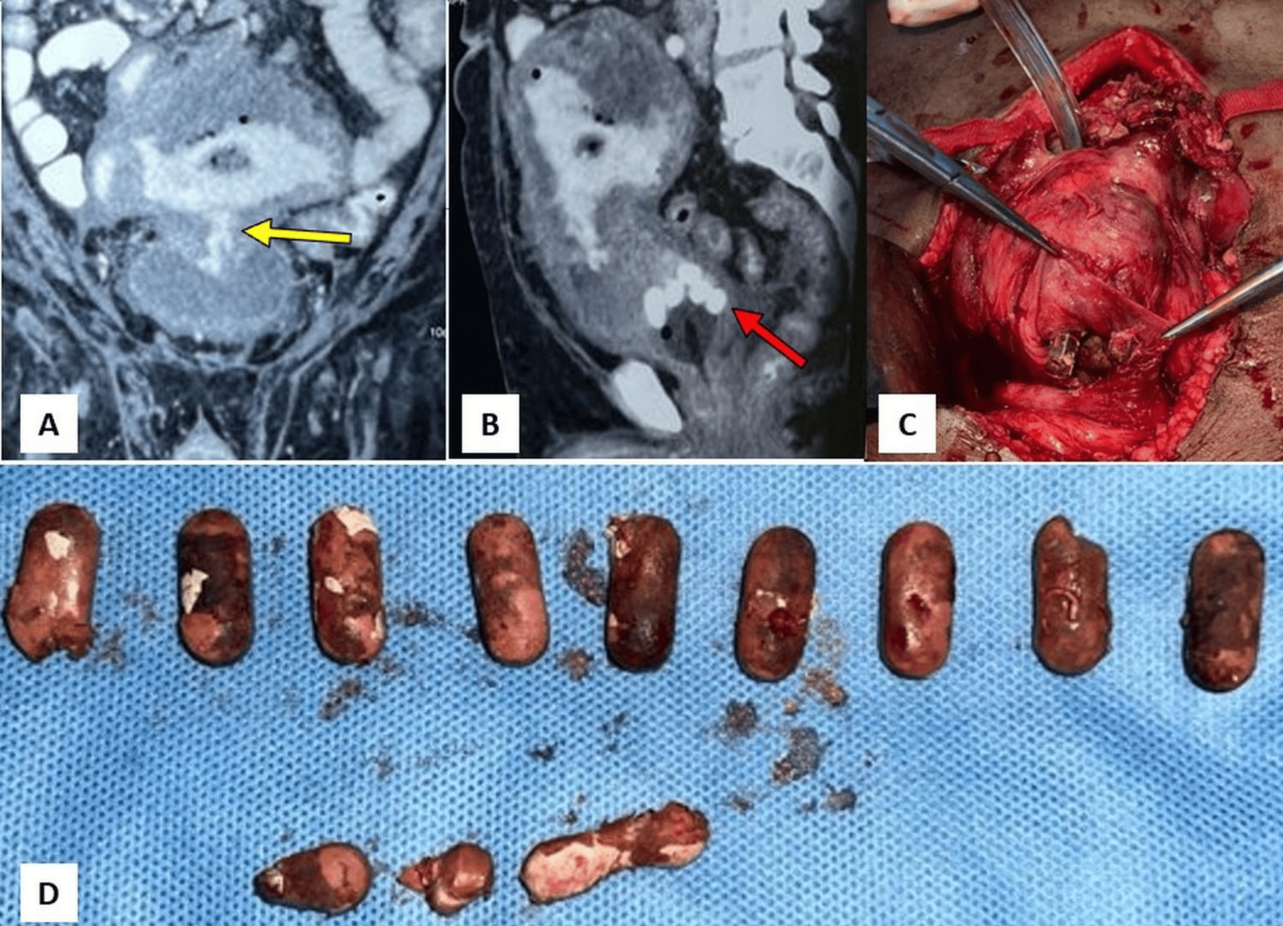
Imaging and surgical retrieval of intravesical foreign bodies migrated via enterovesical fistula in Crohn’s disease (Case 3). (A) Coronal contrast-enhanced CT showing a phlegmonous mass with fistulous communication to the bladder (yellow arrow). (B) Sagittal CT revealing multiple hyperdense intravesical foreign bodies (undissolved tablets) mimicking enteroliths (red arrow). (C) Intraoperative image demonstrating the extraction of retained mesalamine tablets through a bladder incision. (D) Multiple undissolved mesalamine tablets (“ghost tablets”) retrieved from the urinary bladder and laid out on a surgical drape.
